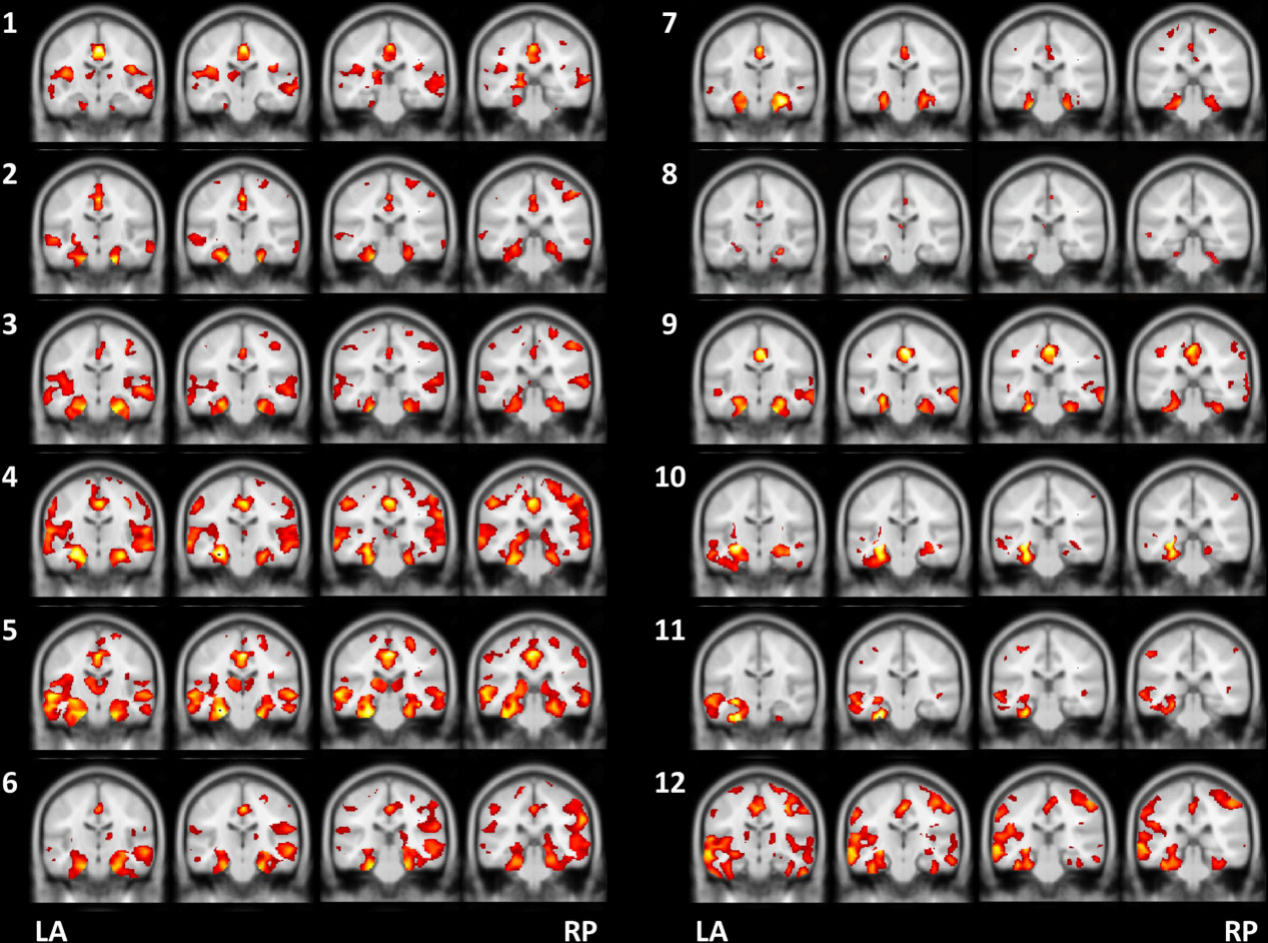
Coronal brain MRI. Analysis of coronal brain MR images with the BAAD revealed patterns of significant atrophy in each of the 12 cases with an unclassified fluent variant of primary progressive aphasia. Case numbers are shown on the left side of each set of images. Cases 1–8: progressive anomic aphasia; Cases 9–11: progressive TCSA; Case 12: progressive Wernicke’s aphasia. BAAD, Brain Anatomical Analysis using Diffeomorphic Anatomical Registration through Exponentiated Lie Algebra; LA, left anterior; RP, right posterior; TCSA, transcortical sensory aphasia.
